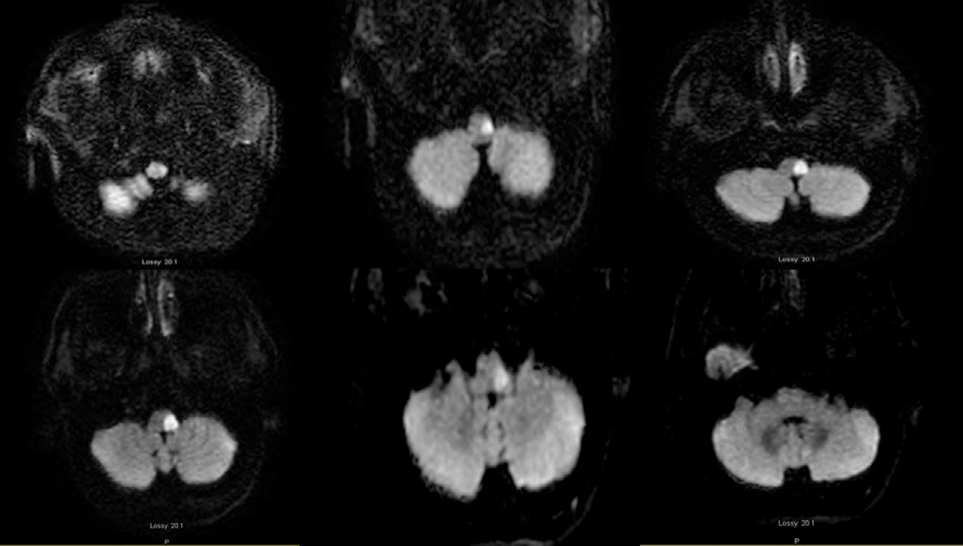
Axial diffusion-weighted image MRI 04-25-2014: serial consecutive MRI sections of the medulla, from left: caudal to right: pontomedullary junction: restricted diffusion is present in the left lateral medulla.
